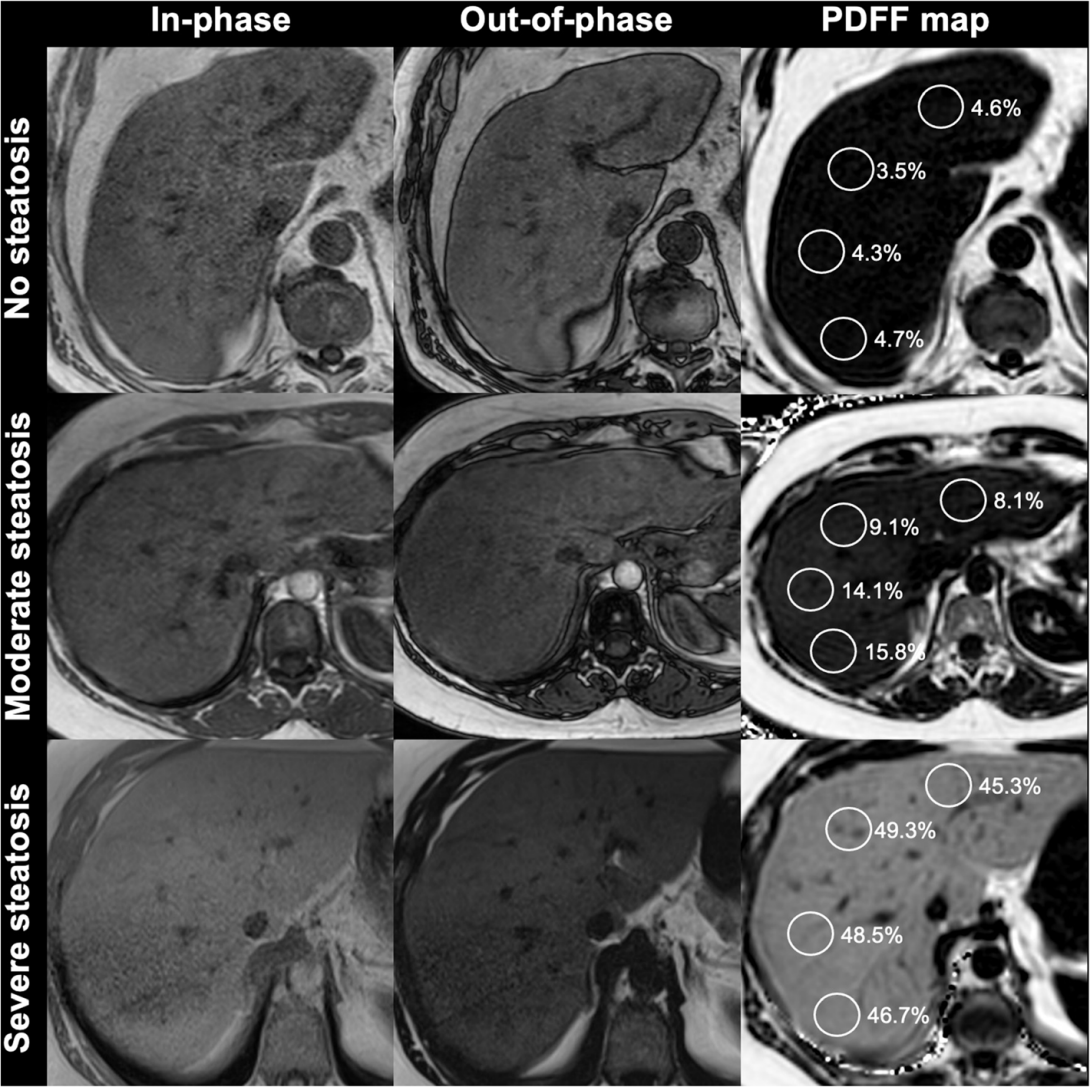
Liver fat detection and quantification in patients with nonalcoholic steatohepatitis undergoing in- and out-of-phase magnetic resonance imaging sequences (first and second columns, respectively), and proton density fat fraction (PDFF, third column). Top row: 78-year-old man without hepatic steatosis. Note the lack of signal drop on out-of-phase image and PDFF of only 3–4%. Middle row: 39-year-old woman with moderate hepatic steatosis. Note the minimal signal drop on out-of-phase image and PDFF from 8 to 16%. Bottom row: 48-year-old man with severe hepatic steatosis. Note the marked signal drop on out-of-phase image and PDFF from 45 to 49%
